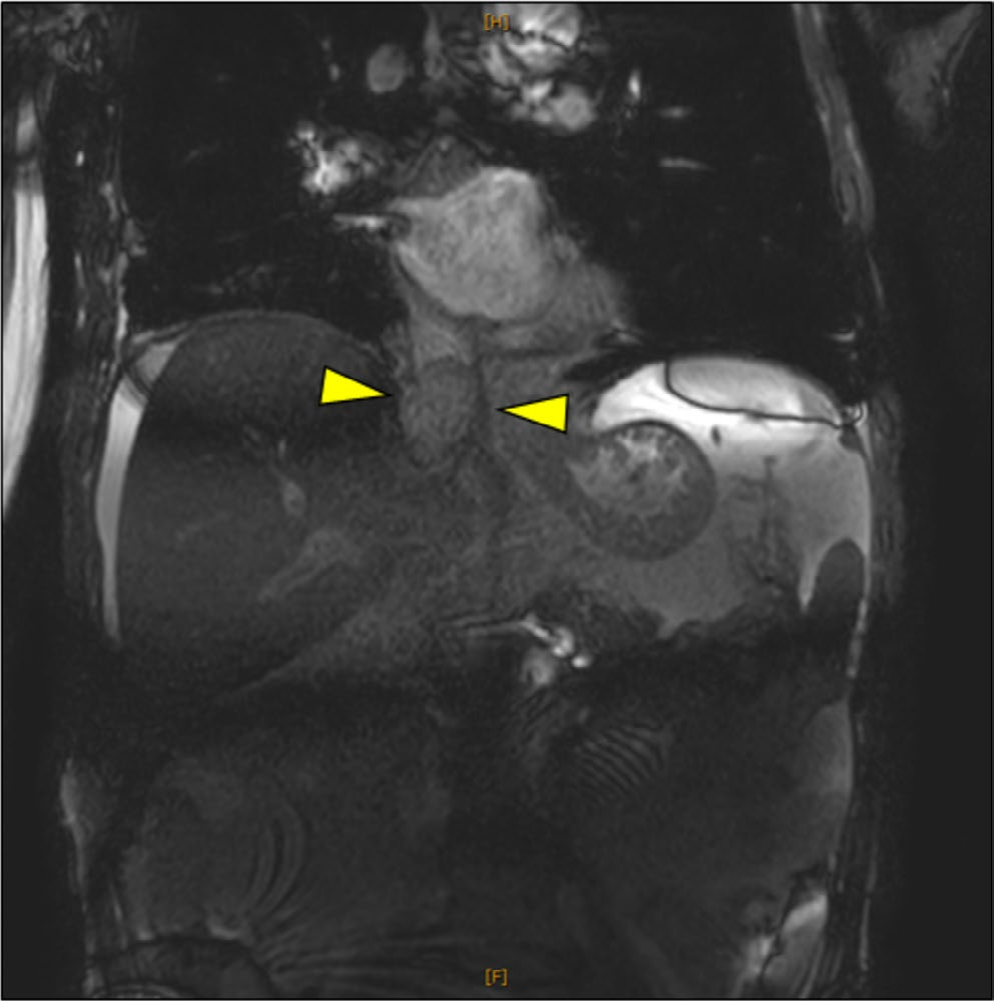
T2‐weighted MRI (coronal view) at the time of recurrence. A mass suggestive of recurrence was identified in the residual IVC, near the hepatic vein inflow.
